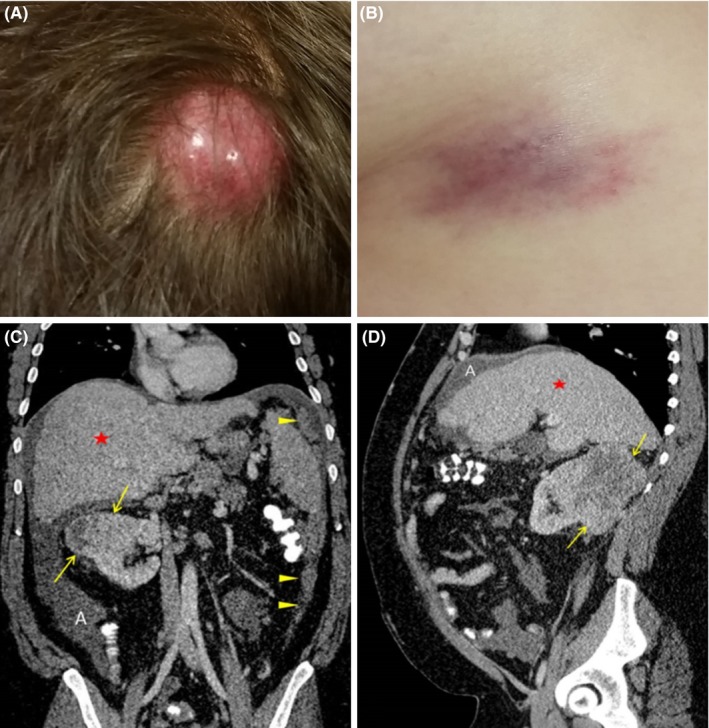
Skin lesions demonstrate, a nodular lesion at scalp (A) and a flat skin lesion at chest wall (B). Multiplanar coronal (C) and sagittal (D) contrast‐enhanced CT of the abdomen demonstrate a heterogeneous solid enhancing mass in the right upper renal pole (arrows) causing anatomic distortion at the renal parenchyma. Malignant peritoneal implants (arrowhead) and ascites (A) are noted. A cirrhotic liver is incidentally seen (*)
